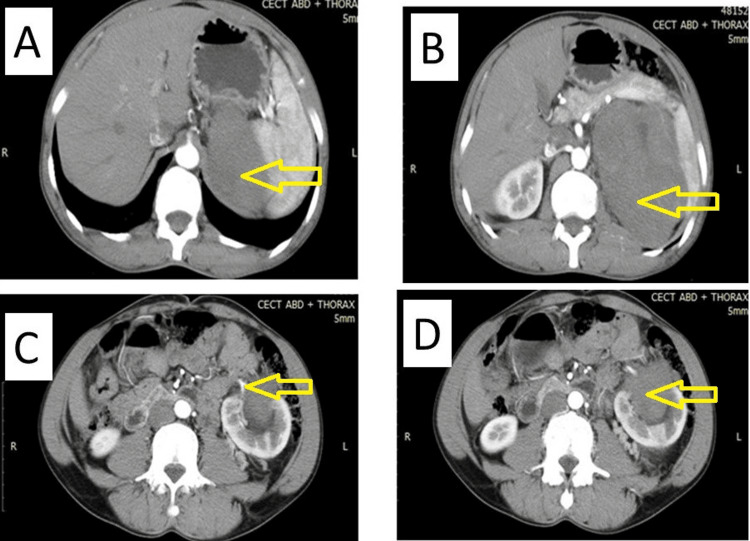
(A) A CT image showing a tumor arising from the left kidney; (B) A tumor displacing the stomach anteriorly, spleen anterolaterally with maintained fat planes; (C) Feeding artery noted arising from the left renal artery; (D) Exophytic mass protruding out from the renal pelvis CT: computed tomograhy
